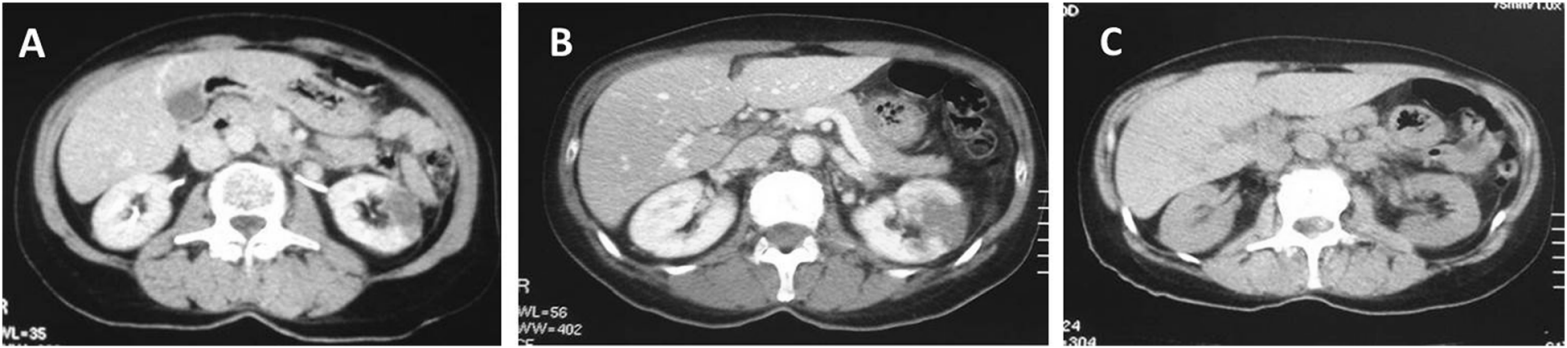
Computed tomography imaging. The first computed tomography. An ischemic area in the left kidney (A). The computed tomography a few days later. An increasing of left renal ischemia tissue and a novel ischemia area in the right kidney (B). The follow-up computed tomography after one year. A reduction of ischemic areas in both kidneys (C).
